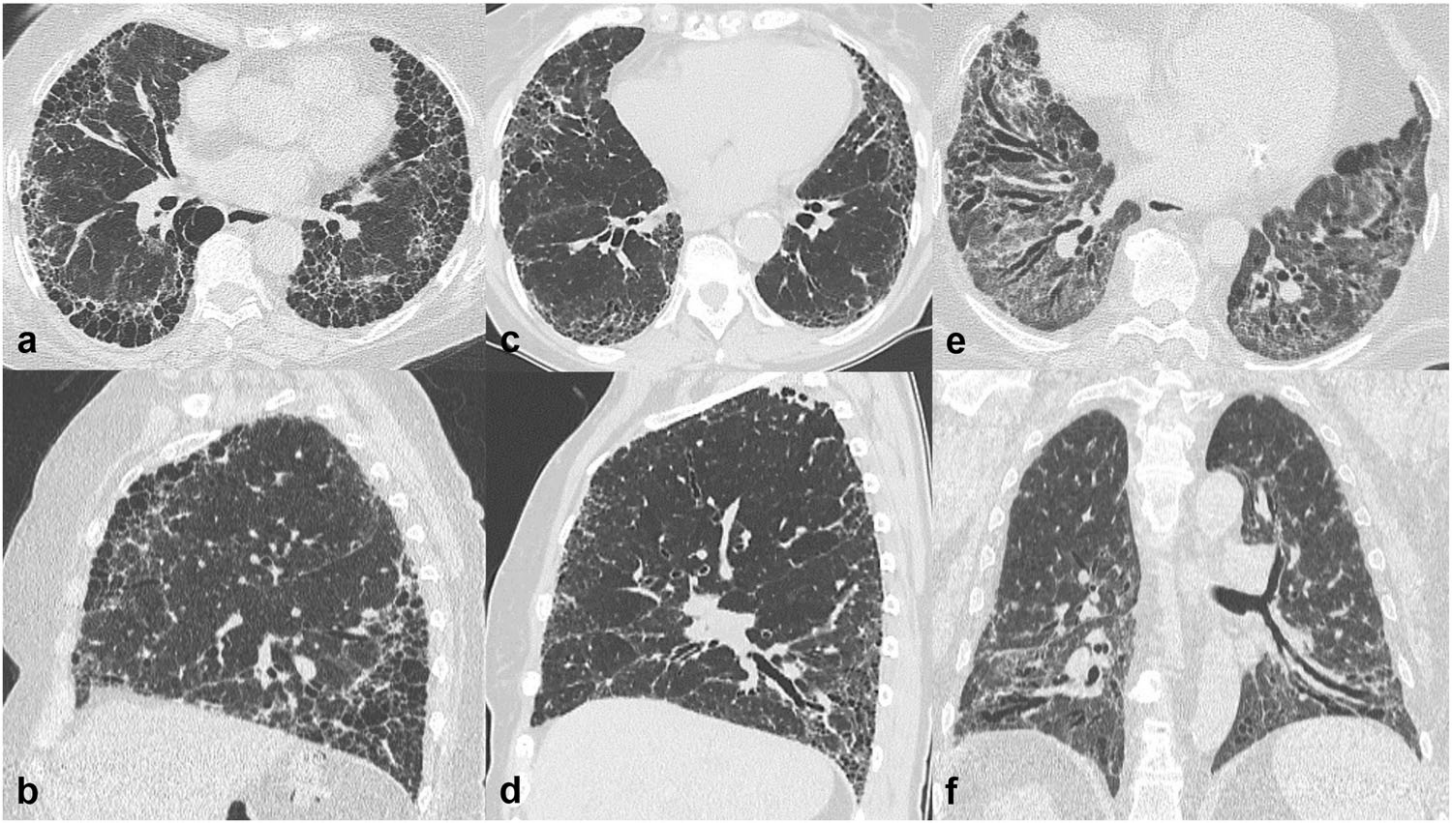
Computed tomography (CT) patterns of fibrotic lung diseases with mid-lower zone predominant distribution. Axial and sagittal CT images showing a usual interstitial pneumonia (UIP) pattern, characterised by honeycombing and reticular pattern with (or without) traction bronchiectasis, and a typical peripheral predominance in the lower lobes but also in the anterior region of the upper lobes (helical distribution) (a, b). Axial and sagittal CT images depicting a probable UIP pattern, characterised by a reticular pattern with distal traction bronchiectasis, with the same distribution as typical UIP but lacking honeycombing (c, d). Axial and coronal CT images of a fibrotic nonspecific interstitial pneumonia (fNSIP), with reticular pattern and traction bronchiectasis showing a typical peribronchovascular distribution. Note the volume reduction of the lower lobes (e, f)
